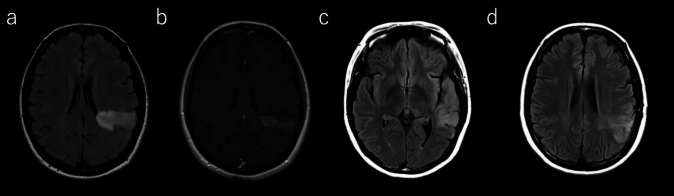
Representative case of a tumefactive lesion. Brain magnetic resonance images from case 39. Initial Fluid-attenuated inversion recovery (a) and T1-weighted gadolinium-enhanced image (b) show a large tumefactive lesion involving the left white matter and adjacent cortex. Six months later, the lesion regressed spontaneously. At 2-year follow-up (c, d), a new focal edematous lesion appeared in the left temporal lobe (c) without gadolinium enhancement. After treatment with intravenous corticosteroids, plasma exchange, and intravenous immunoglobulin, the lesion resolved
